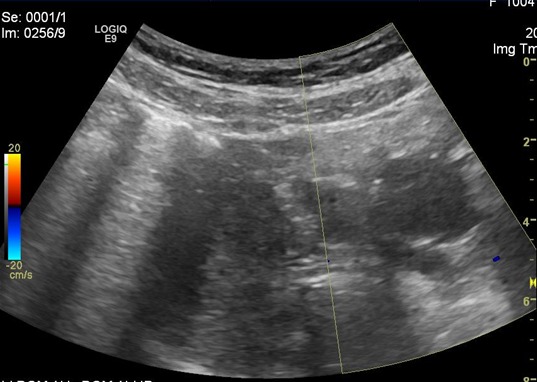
Absence de flux au Doppler couleur au niveau de l'ovaire droit
